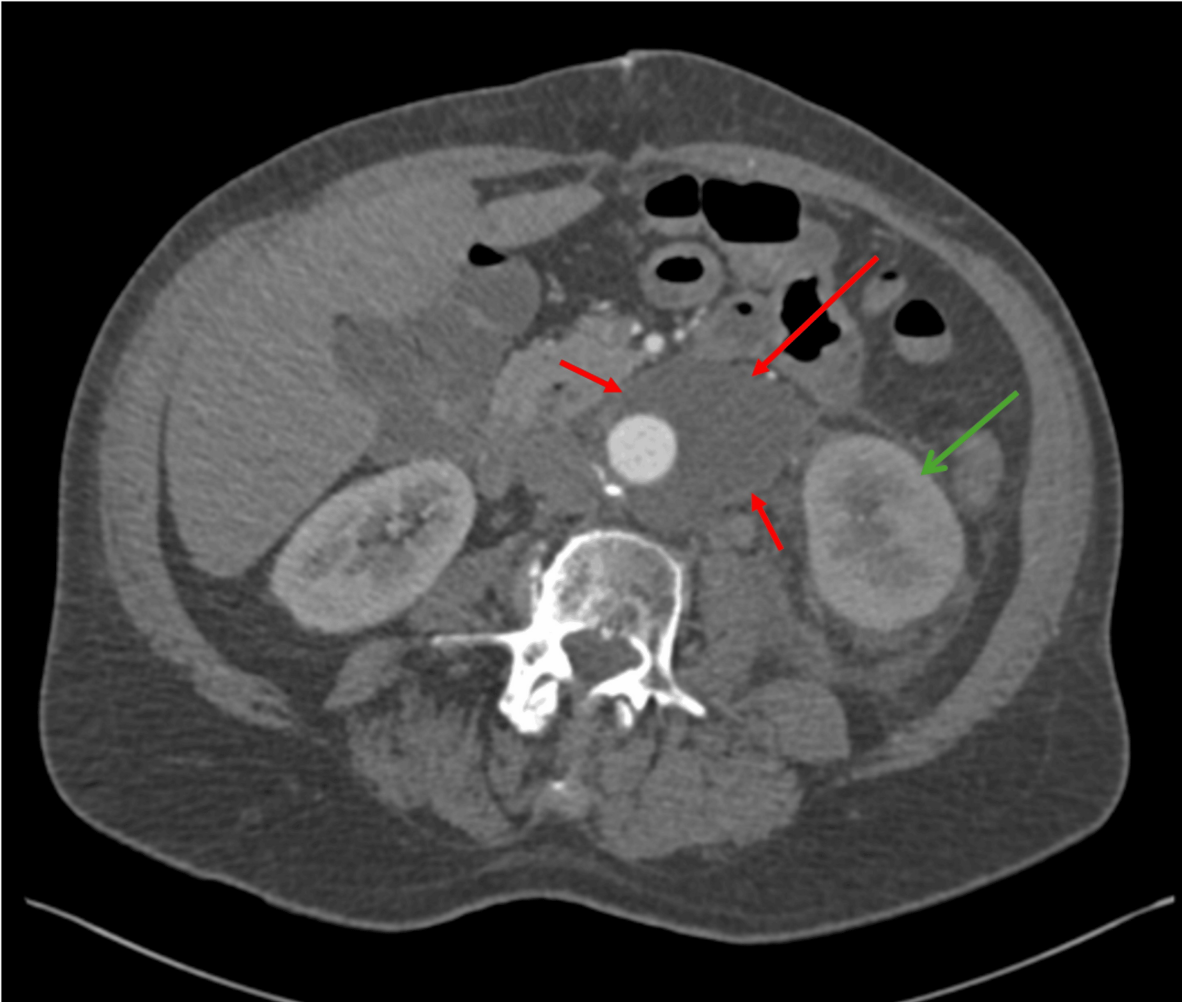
Axial CTA of the abdomen and pelvis with IV contrast (arterial phase) demonstrates retroperitoneal rim-enhancing collection encasing the aortic graft (red arrows) with mild left hydronephrosis (green arrow).
